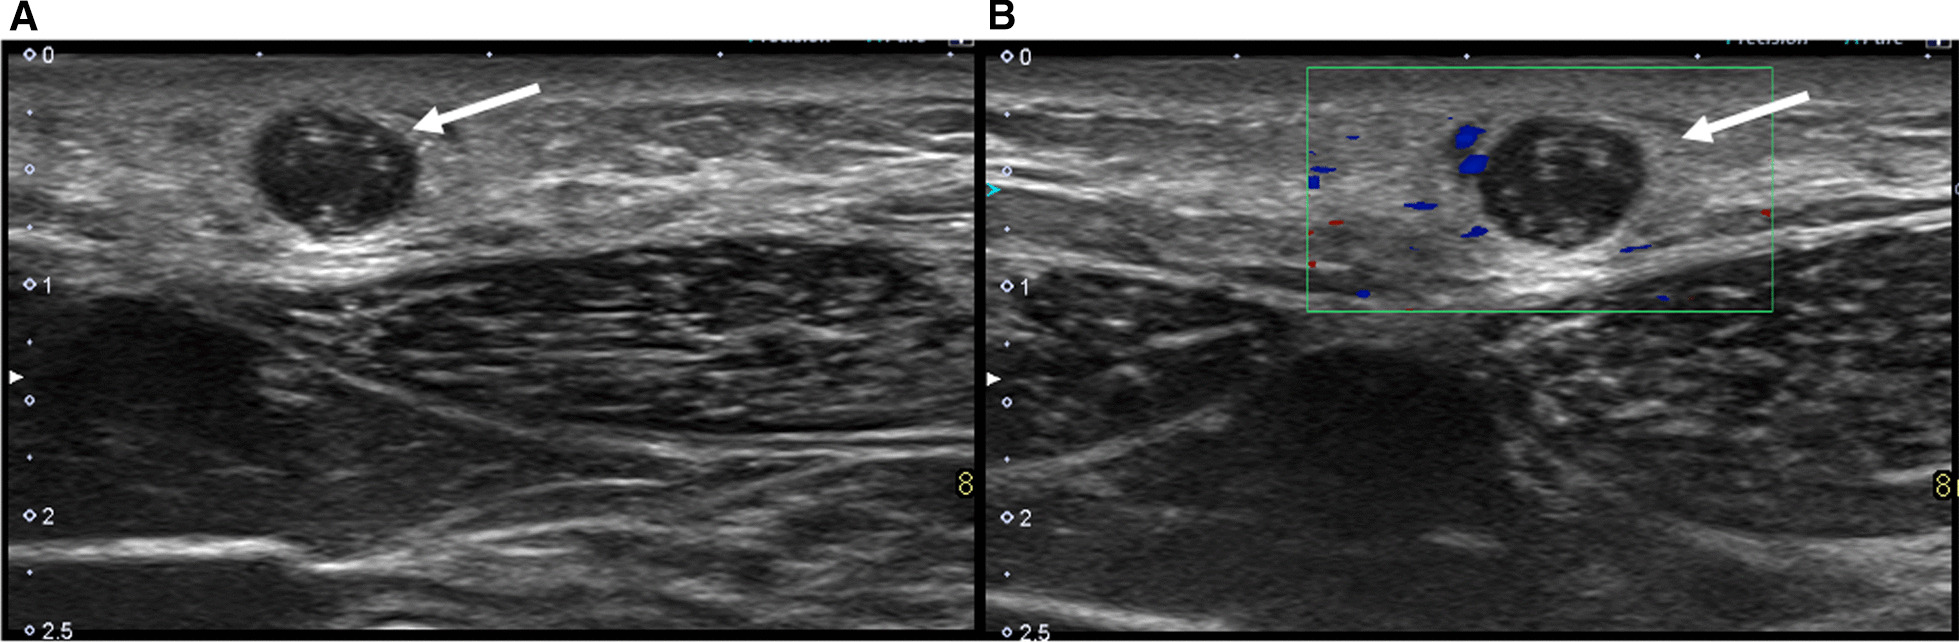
A Ultrasound shows a cystic structure with a diameter of circa 8 mm and an internal tubular serpiginous structure with parallel echogenic lines (arrow). During the investigation, there was active twirling movement of the serpentine structure. B Doppler ultrasonography showed no significant vascularization of the lesion
